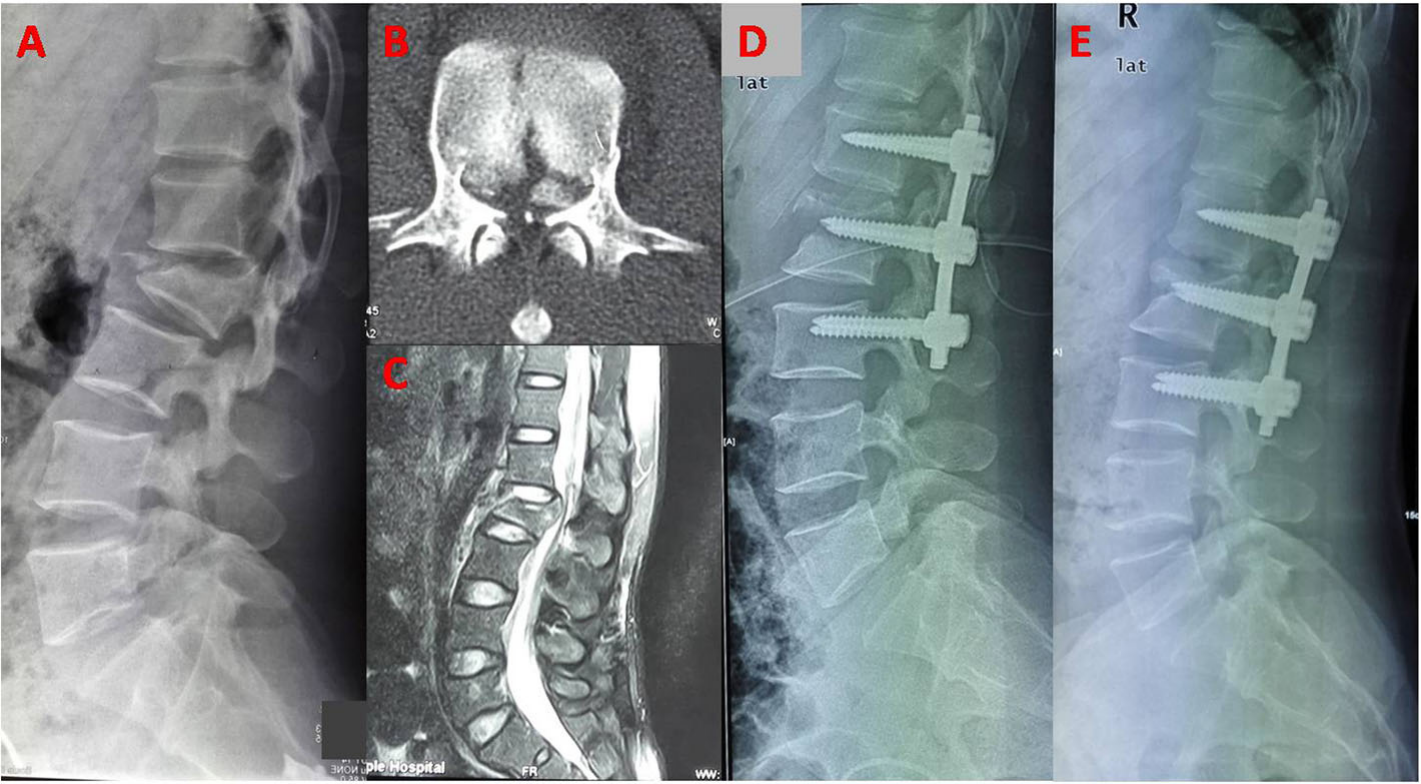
a. A 35-year-old man with an L2 burst fracture; TLICS score is 6 points. b. Axial computed tomography image shows comminution and canal encroachment. c. Sagittal MRI shows PLC injury. (D)Two-level posterior fixation was done from L1 to L3. d. X-ray shows minimal loss of correction at 27 months after surgery
